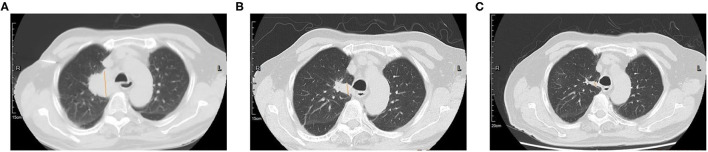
Computed tomography (CT) scan of the primary lung lesion before and after crizotinib treatment. (A) In November 2020, the tumor size was 55 × 43 mm; (B) In January 2021, the tumor size was 26 × 17 mm; (C) In May 2021, the tumor size was 27 × 9 mm.
